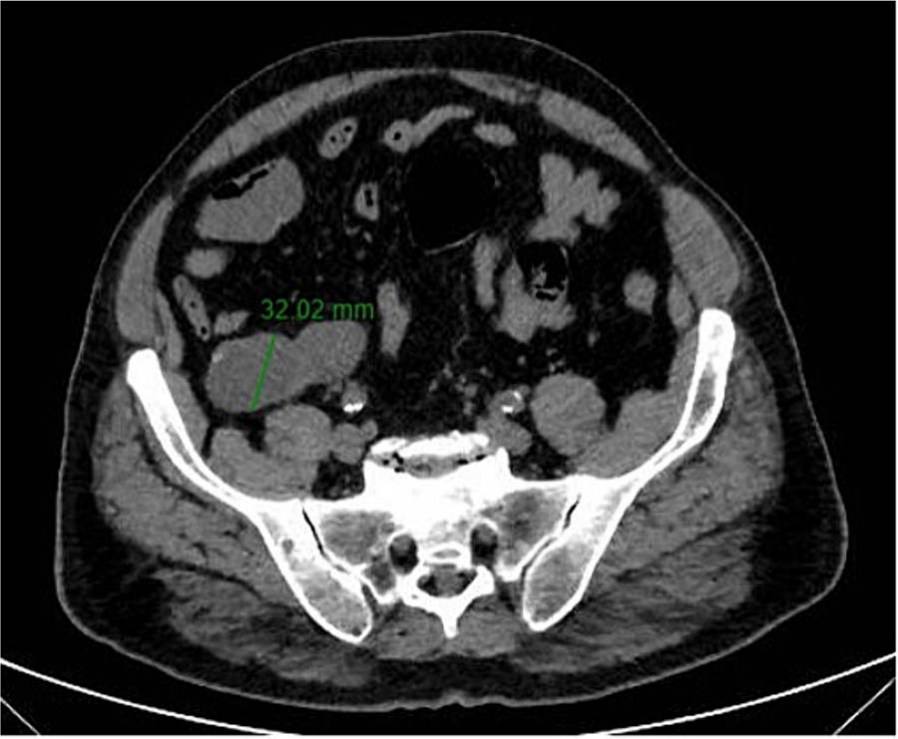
Axial CT-scan highlighting the AM.
